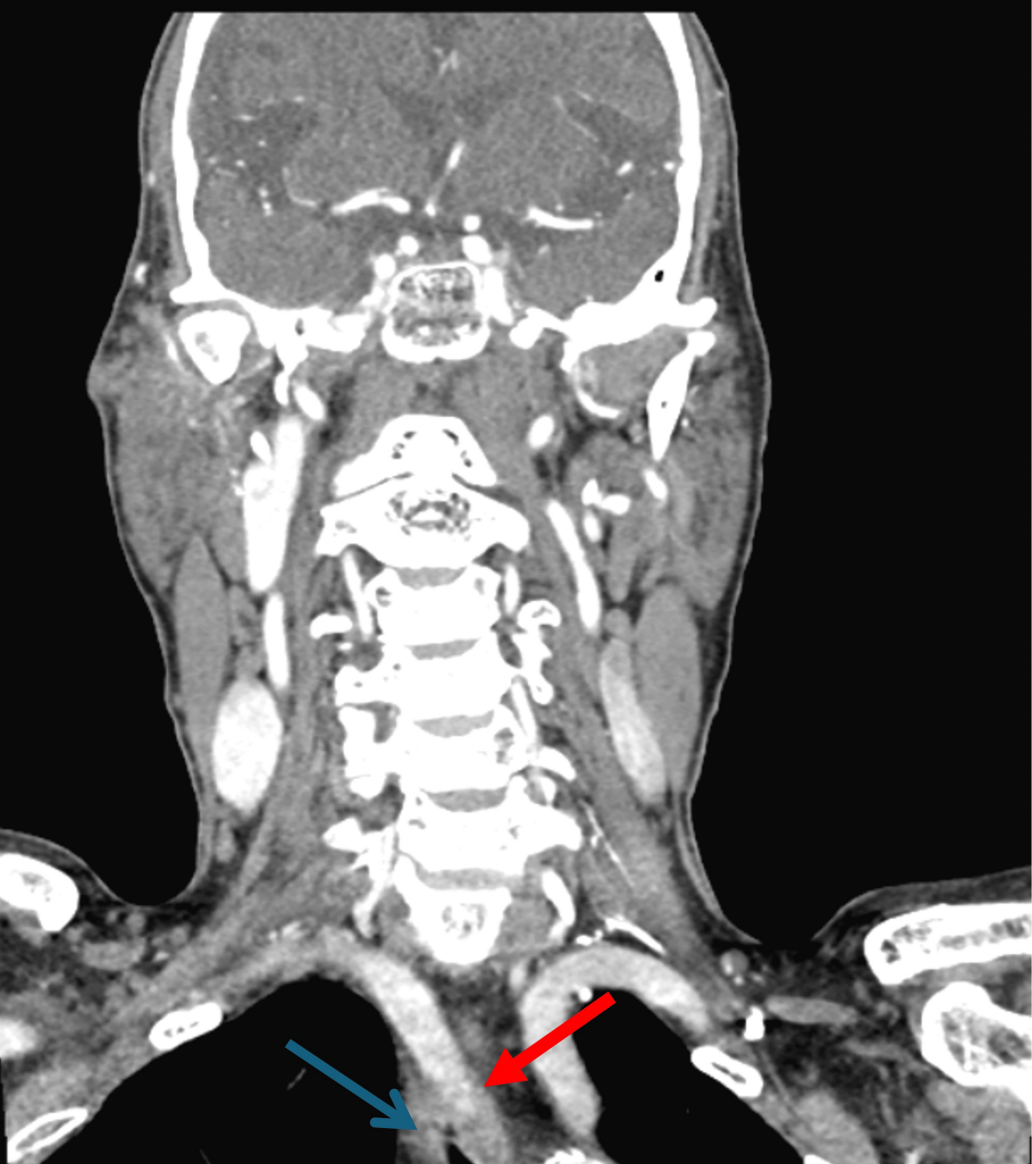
Coronal view of the CT scan of the head and neck with the aberrant right subclavian artery (red arrow) overriding the esophagus (blue arrow).
